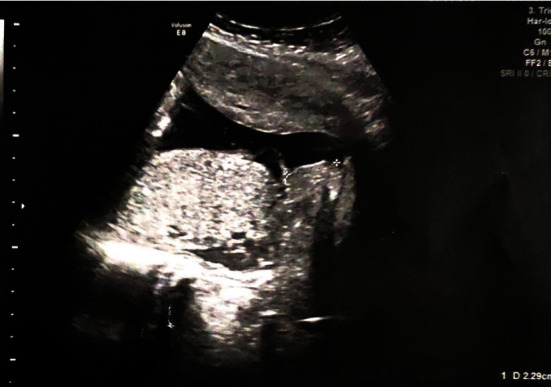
Transvaginal ultrasound indicates the leading edge of placenta at 2.29 cm from the internal os on the day the woman presented at the hospital.
